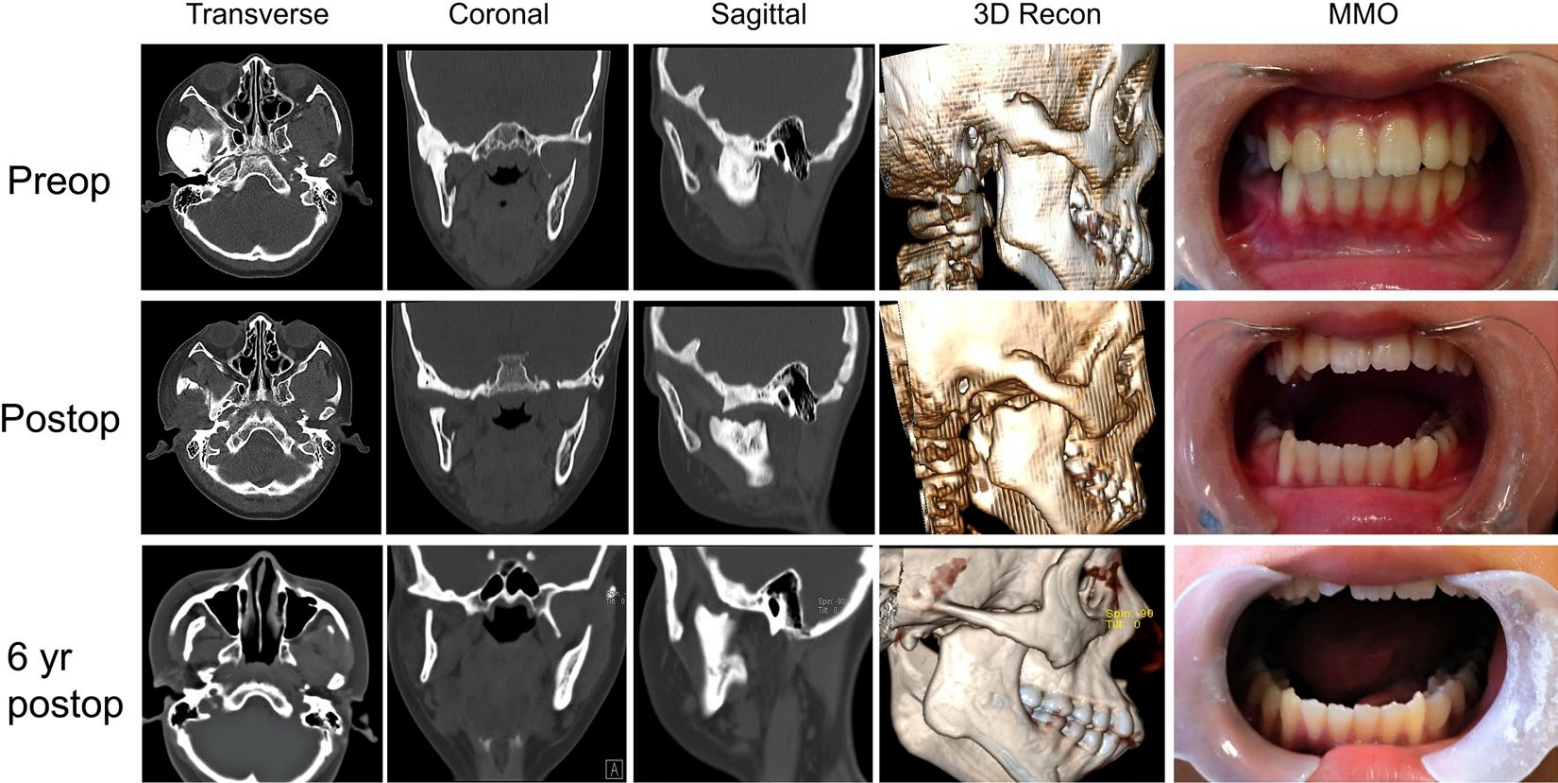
Preoperative, postoperative and 6 years postoperative follow-up CT scan and MMO showed a substantial reduction of the ankylotic block and stable improvement of MMO. (a) Preoperative transverse CT scan; (b) preoperative coronal CT scan; (c) preoperative sagittal CT scan; (d) preoperative 3D reconstruction of CT scans; (e) preoperative MMO; (f) postoperative transverse CT scan; (g) postoperative coronal CT scan; (h) postoperative preoperative sagittal CT scan; (i) postoperative 3D reconstruction of CT scans; (j) postoperative MMO; (k) 6 years postoperative transverse CT scan; (l) 6 years postoperative coronal CT scan; (m) 6 years postoperative sagittal CT scan; (n) 6 years postoperative 3D reconstruction of CT scans; (o) 6 years postoperative MMO.
